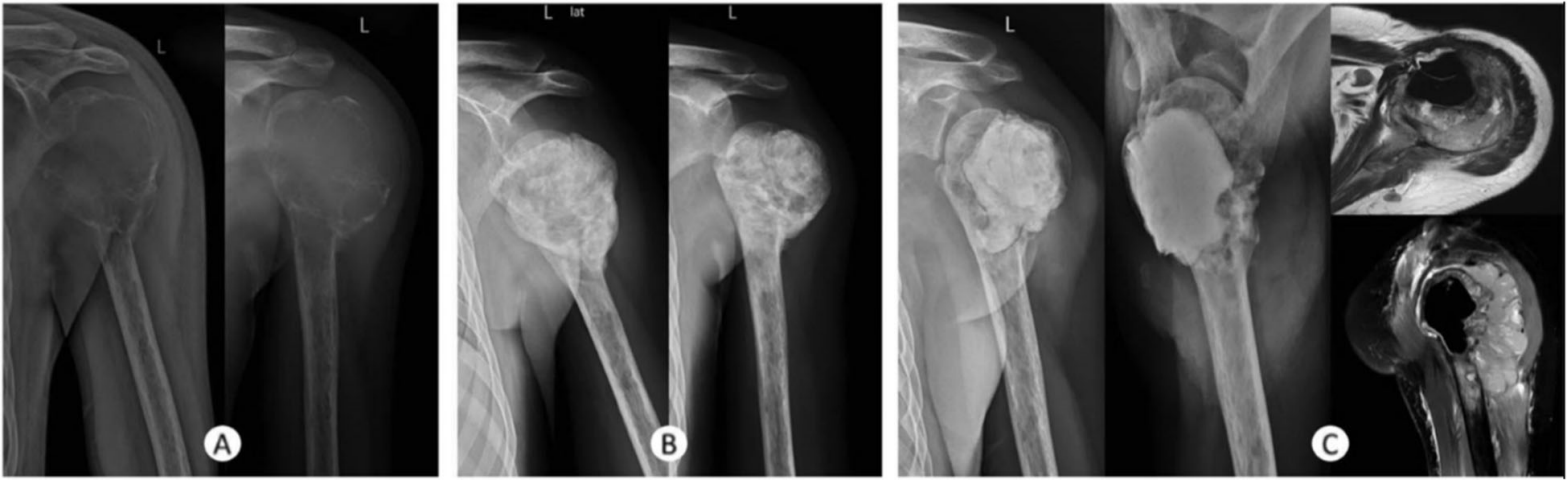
a-c This shows a patient with a proximal humerus GCT with (a) a radiograph at presentation who was treated with eight injections of denosumab, which led to (b) bony shell formation and sclerosis within the lesion after which the patient underwent intralesional surgery for disease clearance. Eight months postoperatively the patient presented with a local recurrence, which as seen on (c) radiography and MRI suggested aggressive malignant transformation. Biopsy confirmed osteosarcoma
